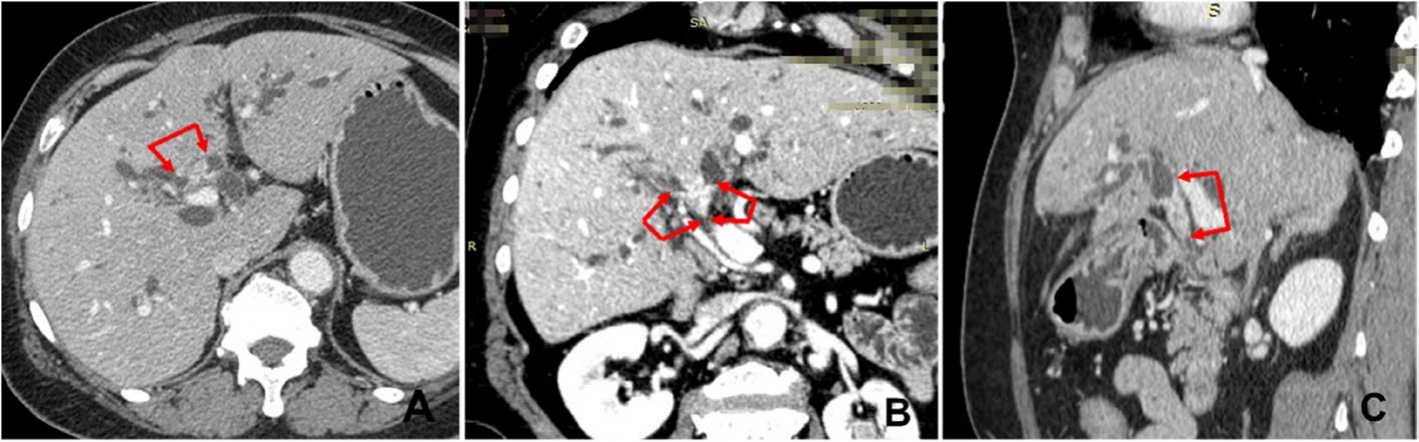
Extent of invasion of hilar cholangiocarcinoma evaluated by multi-slice spiral CT. A multi-slice spiral CT image of hilar cholangiocarcinoma. B Multi-slice spiral CT image of hilar cholangiocarcinoma MPR phase (coronal section). C Multi-slice spiral CT image of hilar cholangiocarcinoma MPR phase (sagittal section)
